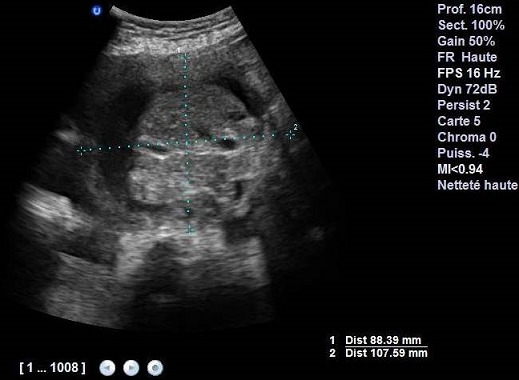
Obstetric ultrasound at 37 weeks containing intestines and part of the liver
